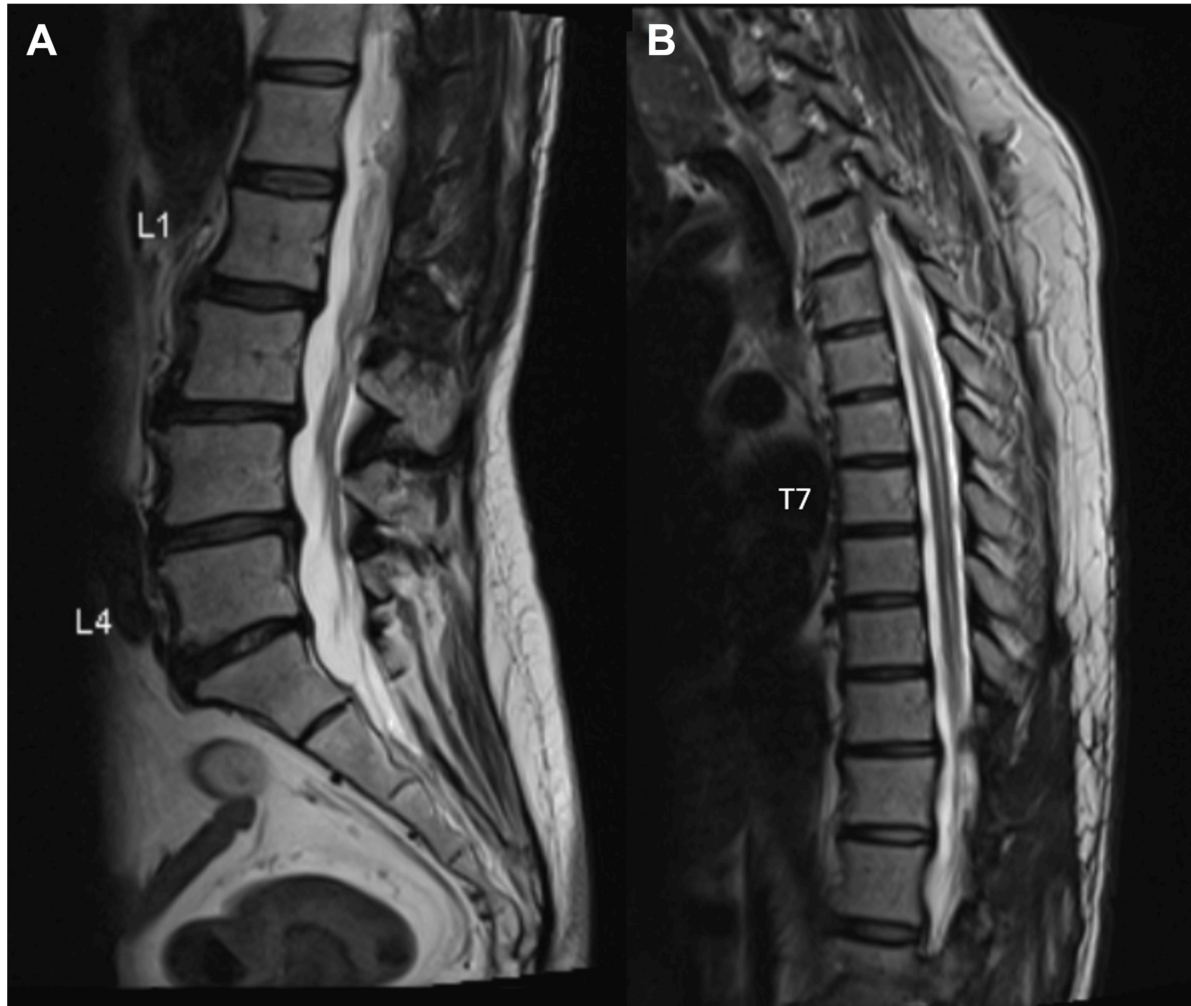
MRI of the spine performed six months after surgery T2-weighted imaging showed significant improvement of the known syrinx. T1-weighted imaging with contrast (not shown) showed a stable residual hemangioblastoma centered at T12 extending to T11 centrally. No new enhancing lesion.
